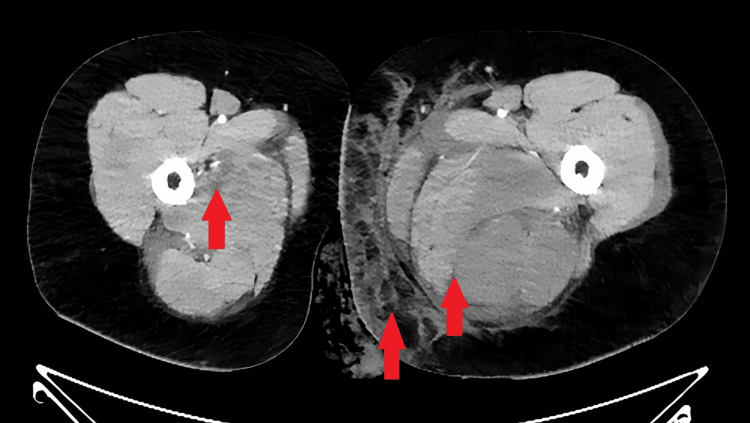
Axial post-contrast CT of the bilateral thighs Axial contrast-enhanced CT shows left greater than right thigh muscle enlargement, muscle edema, and heterogeneous muscle enhancement, most pronounced in the bilateral adductor and posterior thigh compartment musculature. Marked inflammatory changes and edema are also demonstrated within the left medial thigh subcutaneous soft tissues. Arrows mark some of the heterogeneous enhancement and muscle edema in each extremity, as well as inflammatory changes in the subcutaneous tissue of the left thigh.
